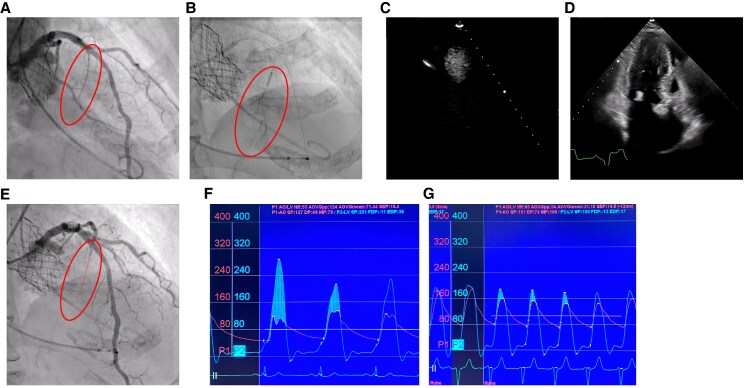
Transcoronary ablation of septal hypertrophy after transcatheter aortic valve implantation as a bail-out procedure. (*A*) Invasive coronary angiography showing the large S1 branch as the transcoronary ablation of septal hypertrophy target. (*B*) Angiography through the over-the-wire balloon showing contrast agent only distal to the balloon and not proximal, validating the proximal occlusion of the S1. (*C* and *D*) Periprocedural transthoracic echocardiography shows SonoVue in the septum for the target zone validation. (*E*) Coronary angiography after transcoronary ablation of septal hypertrophy shows total occlusion of the S1 and TIMI III flow in the left coronary artery. Invasive pressure gradient estimation before (*F*) and after (*G*) transcoronary ablation of septal hypertrophy.
